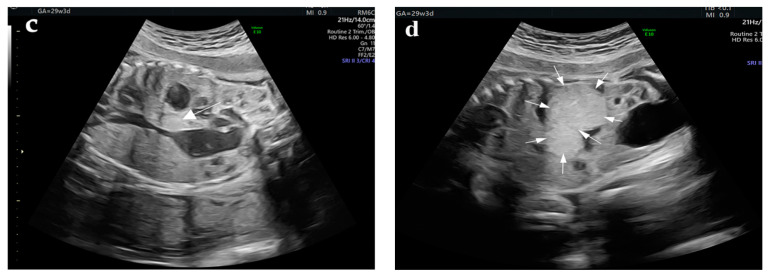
Evolution of ultrasonography findings of a pancreatic tumor in a 25-week-old fetus (a) and at 29 weeks (b,c). (a) Sagittal image shows an echogenic mass (arrows) with a well-differentiated contour in the fetal pancreas. (b) Transversal image revealed an increased echogenic mass at 29 weeks in the fetal pancreas (arrows) compared to the previous examination. (c) Coronal image shows compression of the inferior vena cava by the tumor, with retrograde dilation. (d) Longitudinal image revealed the pancreatic tumor.
